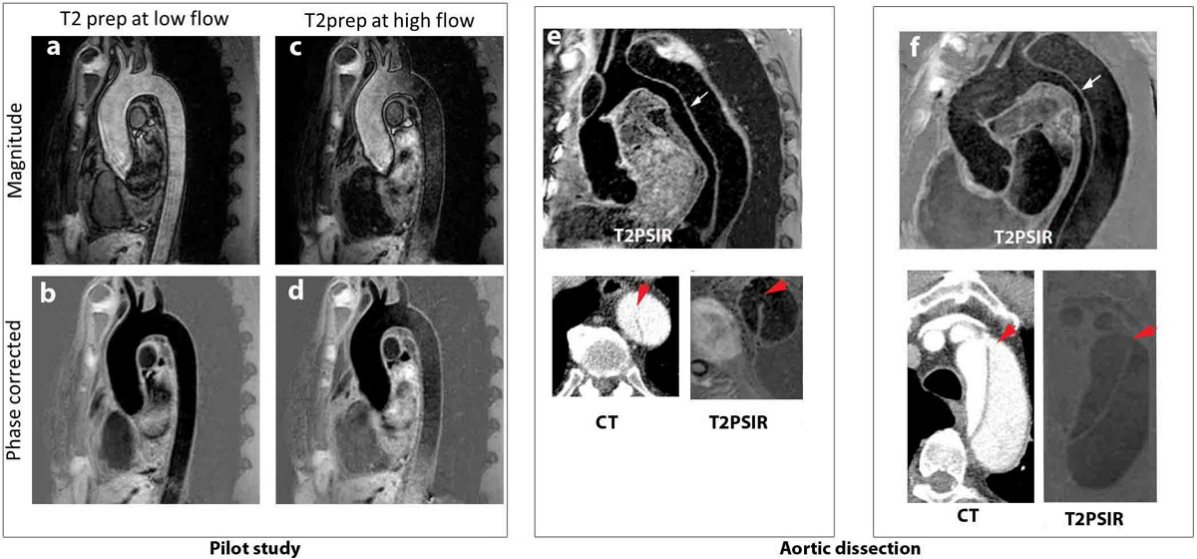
Pilot study results demonstrate flow artifacts if the T2 prep is positioned in a high flow phase (c, d) which are avoided by performing the T2prep in a low flow phase (a, b). Two patients with aortic dissection scanned with the T2PSIR sequence, which shows the intimal tear and flap clearly (e, f). Corresponding CT slices showing the tear and intimal flap are included for comparison.
